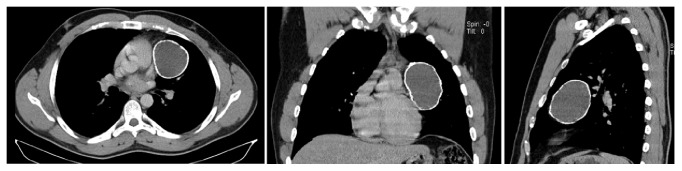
Thoracic CT showing left anterolateral mediastinal mass with total parietal calcification (mediastinal window) [right: horizontal section, middle: frontal section, and left: sagittal section] (Patient2).
